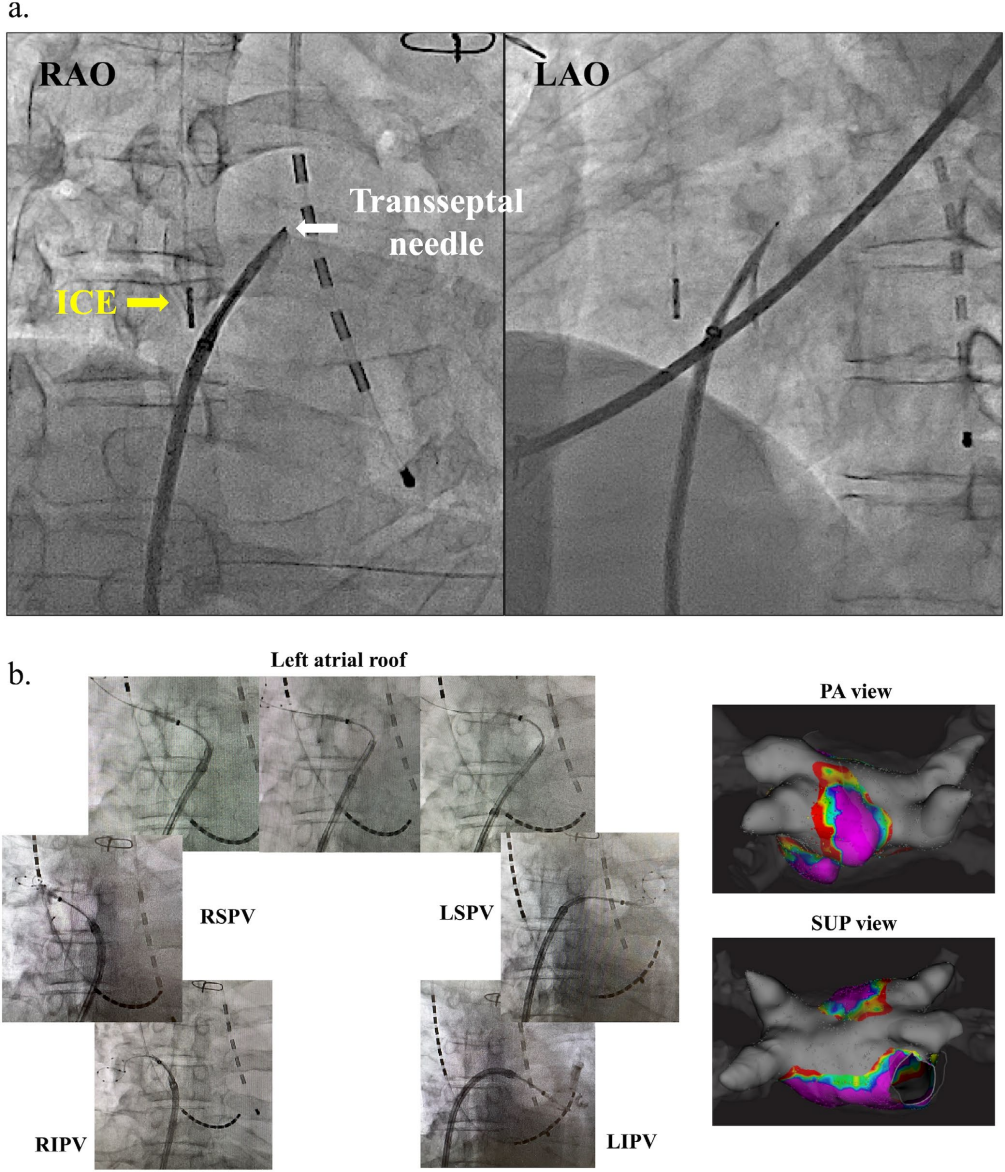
Atrial septal puncture and ablation procedure. (a) An atrial septal puncture was performed under the guidance of an intracardiac echocardiography (ICE) catheter, which was inserted through the right jugular vein. (b) PVI and left atrial roof‐line ablation were successfully carried out using a cryoballoon. Post‐ablation images recorded with electroanatomical mapping are shown. The voltage map indicates the completion of PVI and scarring of the left atrial roof. The purple regions represent a voltage greater than 0.5 mV, while the gray regions indicate a voltage less than 0.1 mV. ICE, intracardiac echocardiography; LAO, left anterior oblique; LIPV, left inferior pulmonary vein; LSPV, left superior pulmonary vein; PA, posterior–anterior; PVI, pulmonary vein isolation; RAO, right anterior oblique; RIPV, right inferior pulmonary vein; RSPV, right superior pulmonary vein; SUP, superior.
